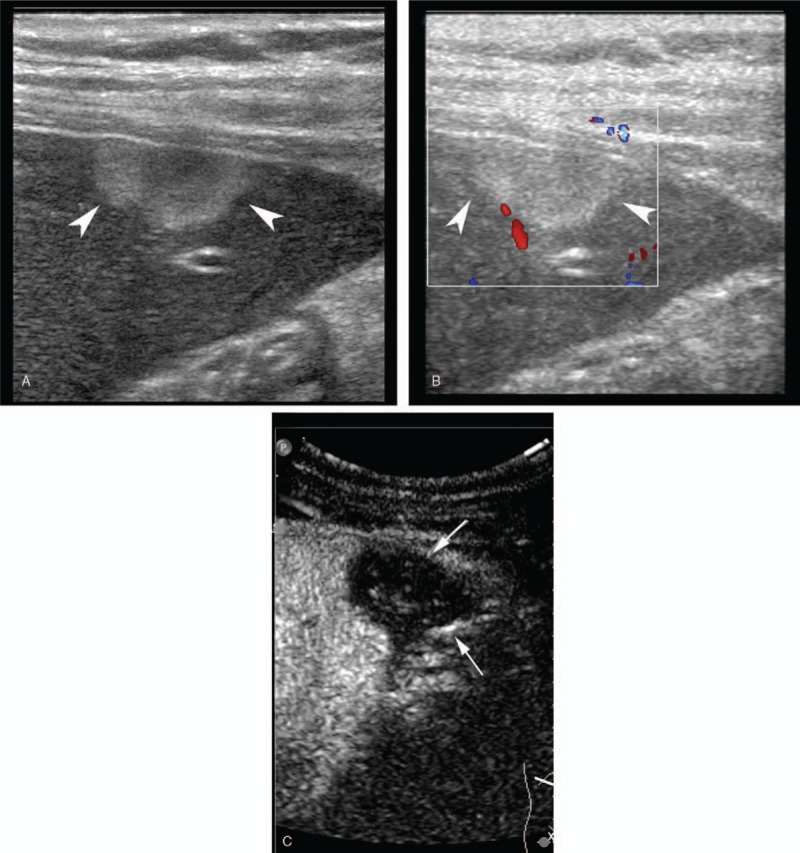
A 40-year-old man with hepatic AE which was misdiagnosed as hepatic hemangioma by US. A: A hyperechoic irregular lesion (arrow head) was found in the left hepatic lobe on US; B: No signal in the lesion (arrow head) was detected using color Doppler mode. C: The lesion (arrow) showed nonhomogeneous hypo-enhancement after contrast agent injection in the late phase by CEUS. CEUS = contrast enhanced ultrasound, US = ultrasound.
